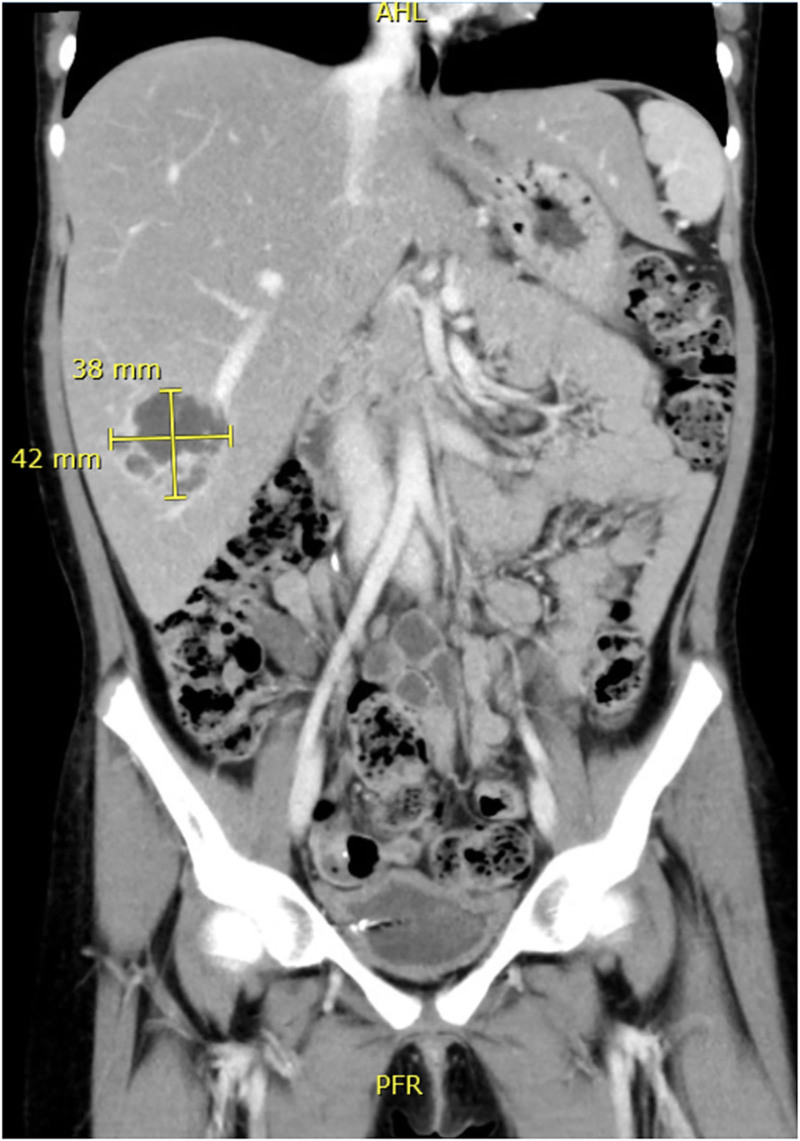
Coronal abdominal CT scan of the abdomen showing 3.8 × 4.2 cm multilocular cystic lesion in liver segment 6. CT = computed tomography.
